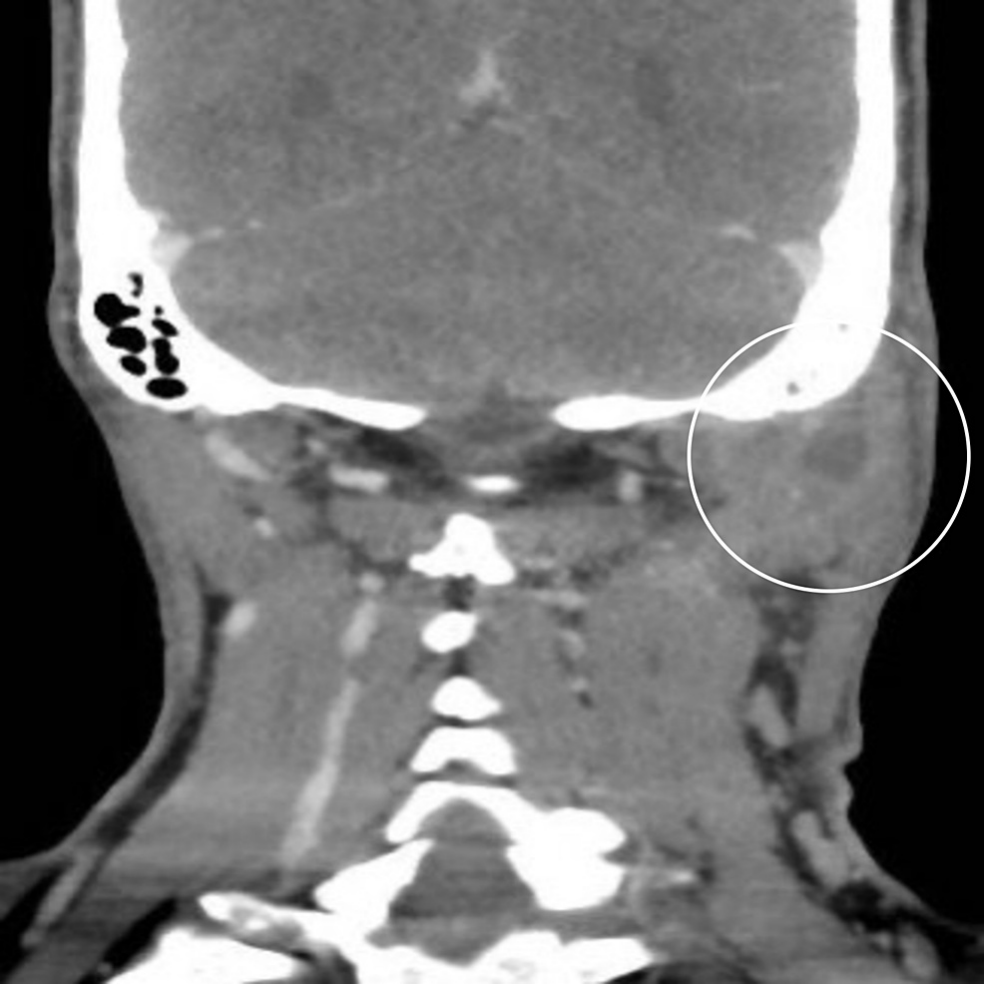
Coronal enhanced CT image of the head and neck showing opacification of the left lateral inferior to the mastoid area with rim enhancement, medial to the sternocleidomastoid muscle, a typical picture commonly seen with Bezold’s abscess.
